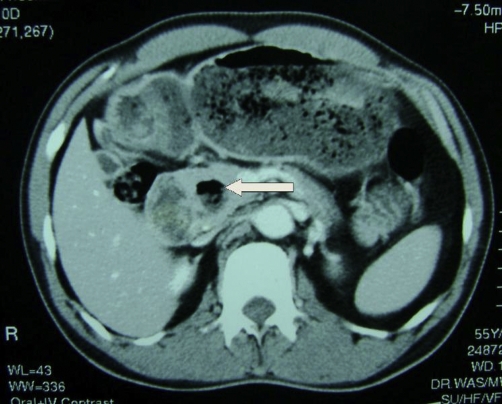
Contrast enhanced CT scan demonstrating the diverticulum (indicated by white arrow) extending towards the head of the pancreas.
